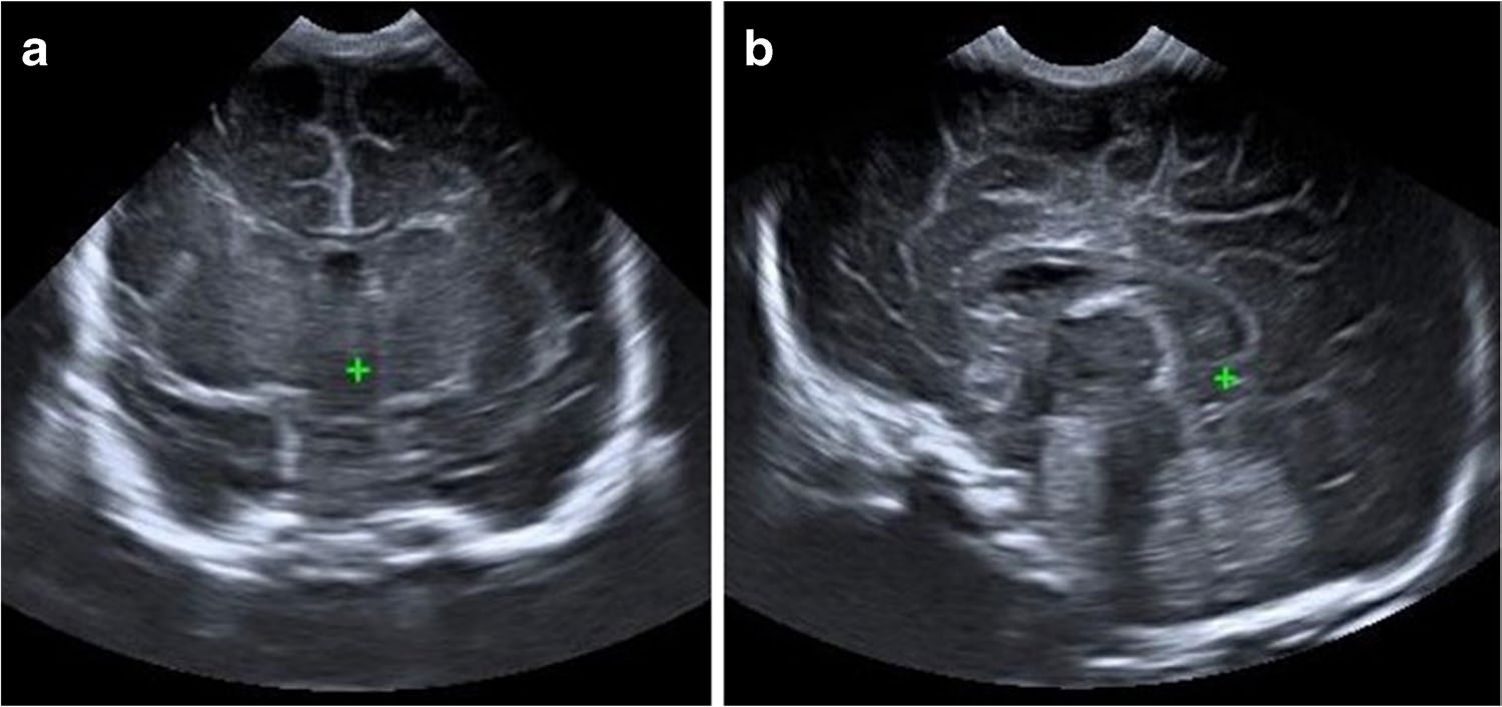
A 41-day-old girl with a normal cranial ultrasound (US). Images obtained in the baseline probe location at the anterior fontanelle for the 3-dimensional US volume acquisition, for the coronal (a) and sagittal (b) planes
